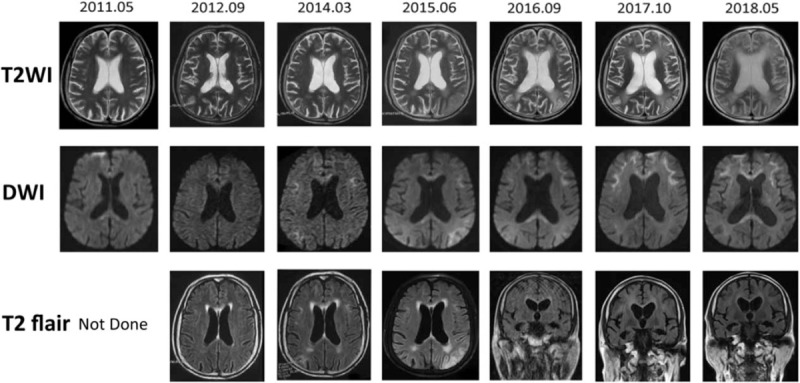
Seven years follow-up of patient 1. The DWI high-intensity signals were observed in the frontal lobes at first and spreading backward along corticomedullary junction. In the image of 5 years after onset, the abnormal DWI signal disappeared. DWI = diffusion-weighted imaging.
